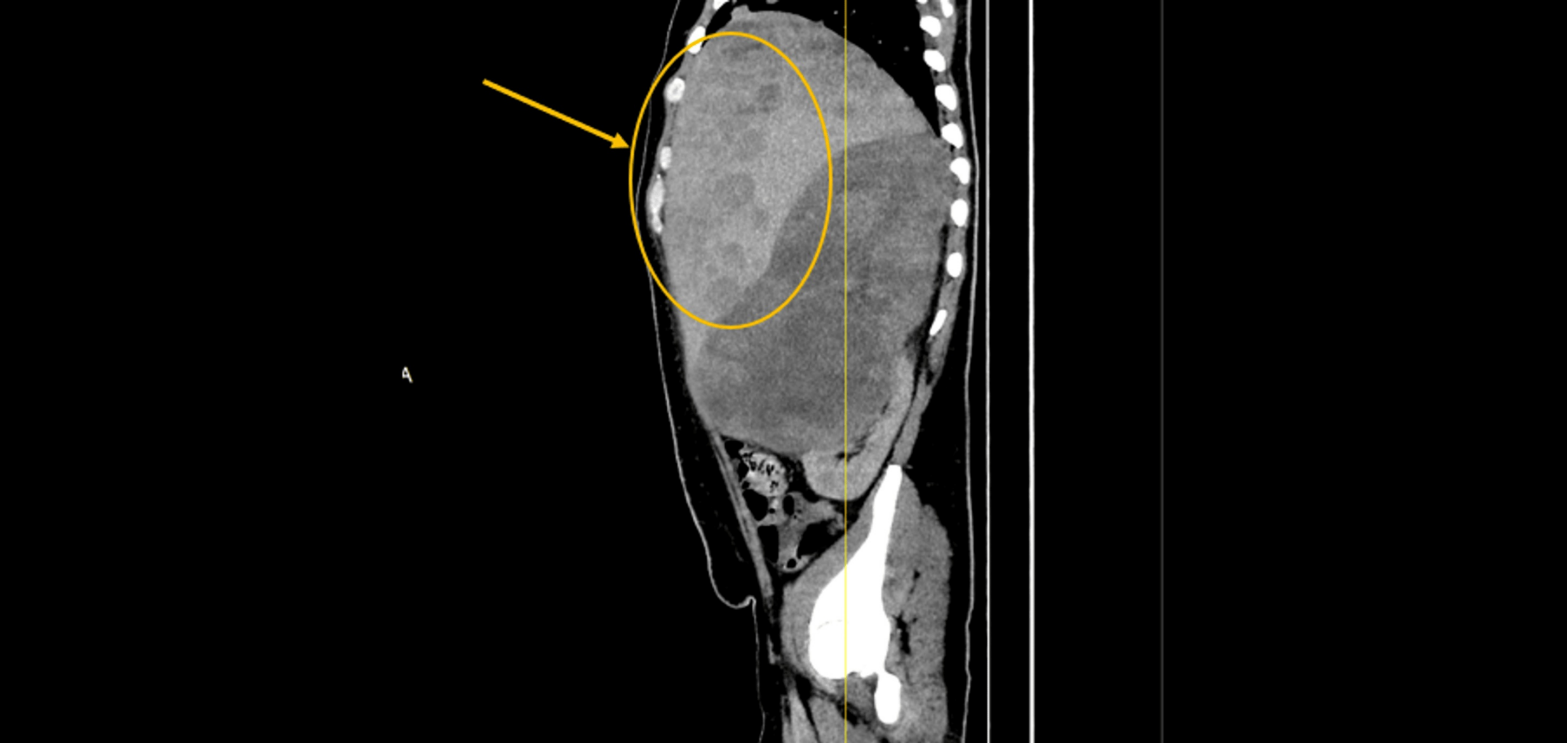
CT scan of the abdomen revealed multiple hypodense liver lesions, measuring up to 3.4 cm, which were consistent with hepatic metastases.
